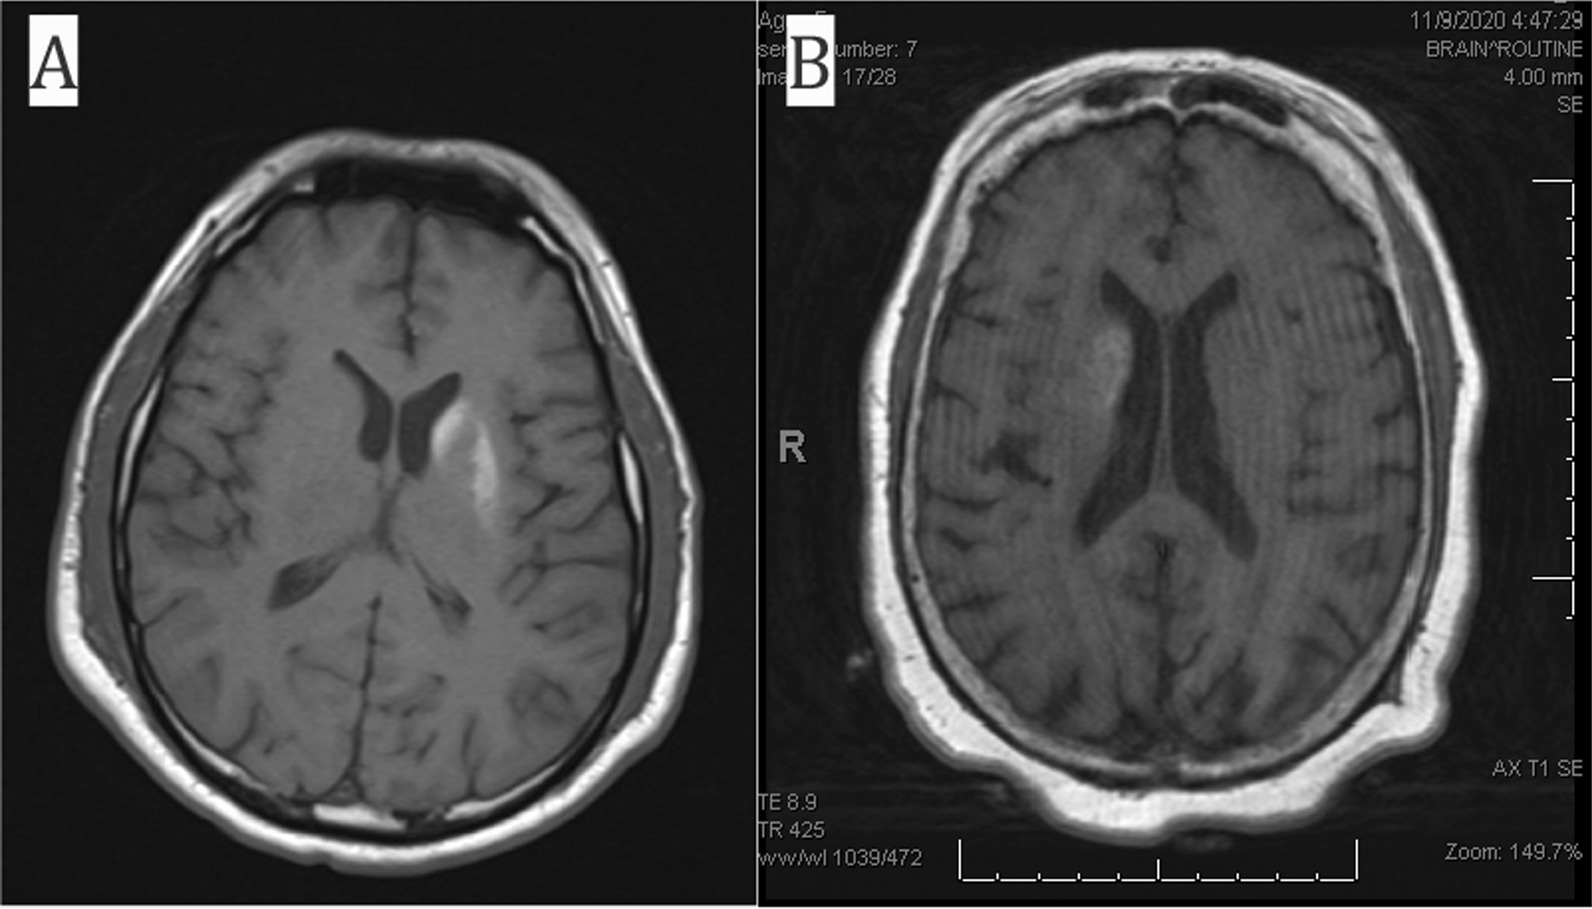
Brain MRI T1-weighted axial images representing basal ganglia lesions secondary to hyperglycemia in HBHC syndrome patients. A Diffuse hyperintensity of the left caudate and lentiform nucleus seen in a 44-year-old Hispanic male from case 1. B Diffuse hyperintensity of the right caudate nucleus seen in a 78-year-old Haitian female from case 2
